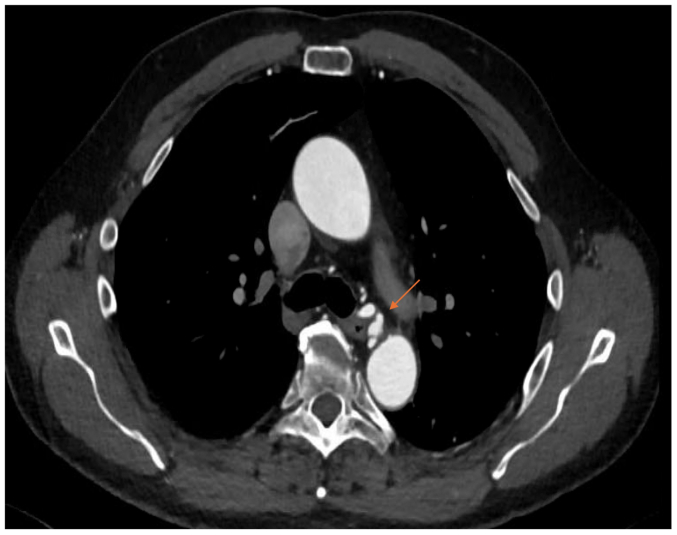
Computed tomography angiogram (CTA) transverse view showing right bronchial artery beading appearance, *orange arrow*.
